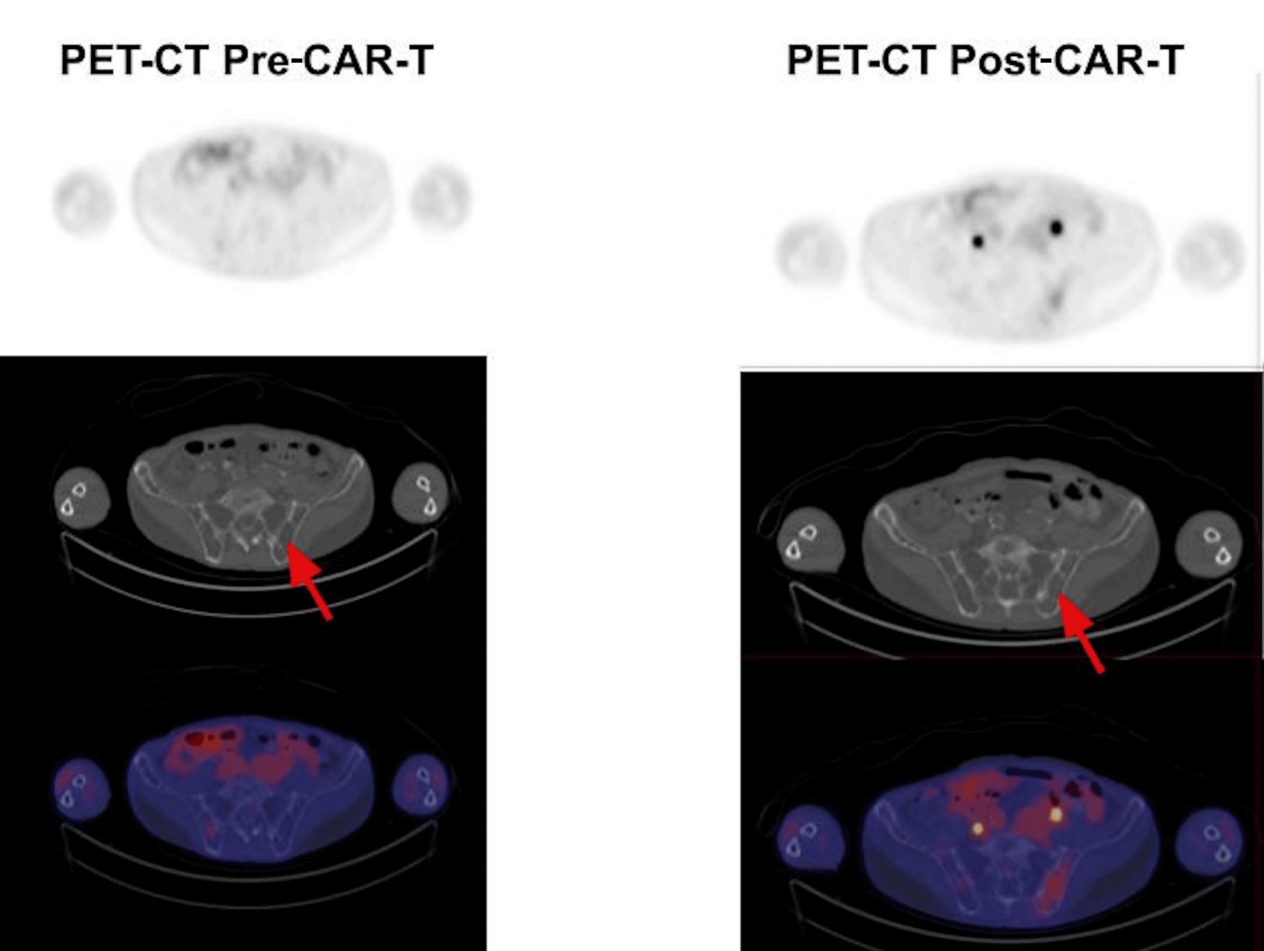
Pre- and post-CAR-T PET-CT scan. No abnormal focal activity observed, all below hepatic SUV. Diffuse lytic and mottled appearance of the osseous structures (red arrow). No focal activity within the osseous structures suggesting metabolic active disease. PET-CT: positron emission tomography-computed tomography; CAR-T: chimeric antigen receptor T-cell; SUV: standardized uptake value
